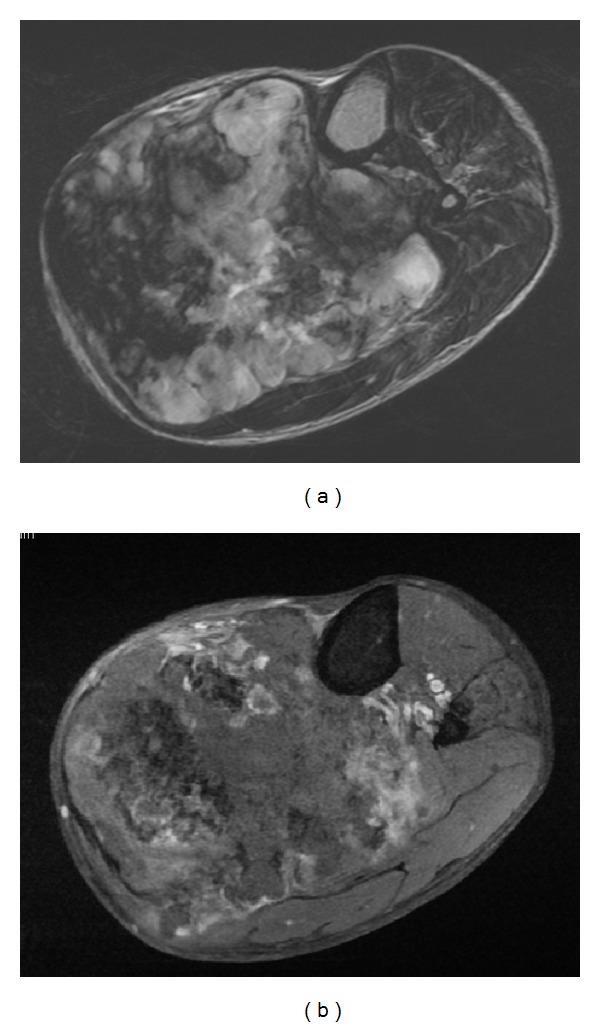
Axial MRI scan of tibia and fibula demonstrating a peripheral dedifferentiated chondrosarcoma with soft-tissue mass and heterogeneous T2 weighted (a) and T1 weighted with contrast (b) enhancement.
